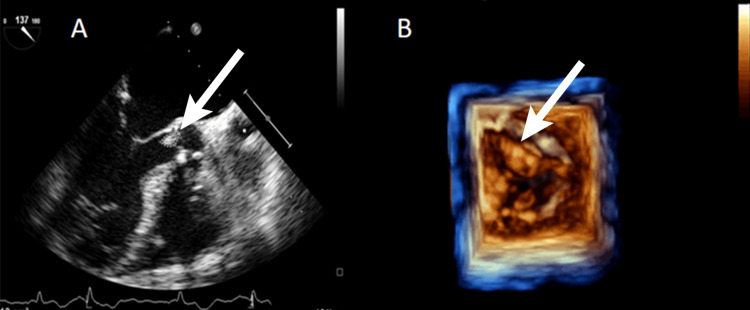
Echocardiogram showing vegetation over the aortic valve. (A) Transesophageal echocardiogram. (B) 3D echocardiogram.
